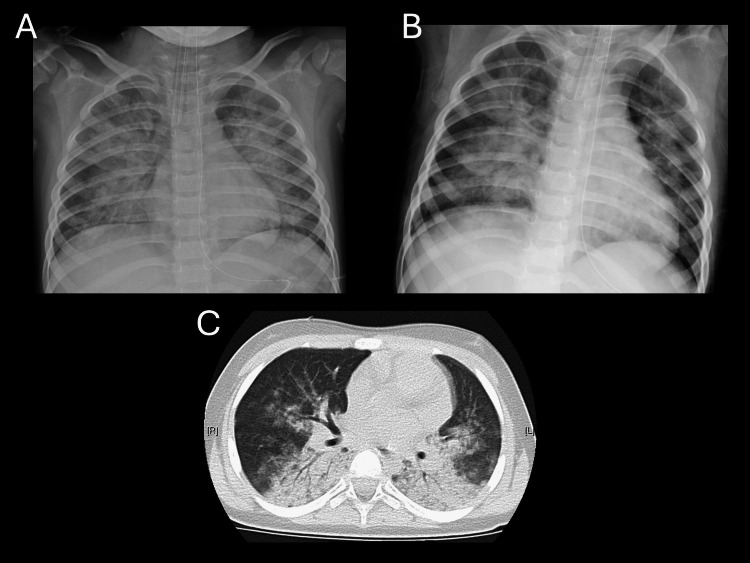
Chest radiographs and chest CT images in case 2 (A) Chest radiograph obtained before prone positioning. (B) Chest radiograph obtained after prone positioning, showing slight improvement in lung radiolucency compared with panel A. (C) Chest CT image obtained before prone positioning, demonstrating extensive dorsal atelectasis. Note: The left side of each image corresponds to the patient’s right side.
